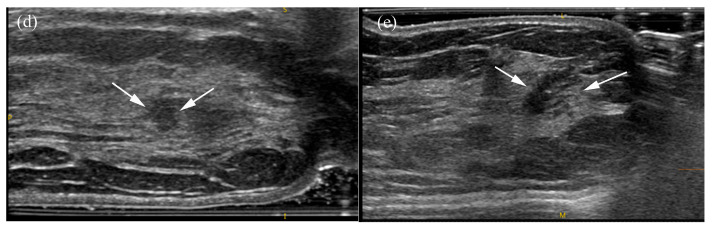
A 66-year-old female patient with screen-detected invasive lobular and ductal carcinoma: (a,b) The malignancy is not visualized on digital breast tomosynthesis (DBT). (c) Hand-held ultrasound reveals a spiculated, irregular, heterogeneous hypoechoic mass (arrows) in the left 12 o’clock area. (d,e) MammouS-N transverse images in craniocaudal (d) and mediolateral oblique (e) views demonstrate an indistinct, irregular, heterogeneous hypoechoic mass (arrows) in the central upper area of the left breast. S = superior, I = inferior, and M = medial.
